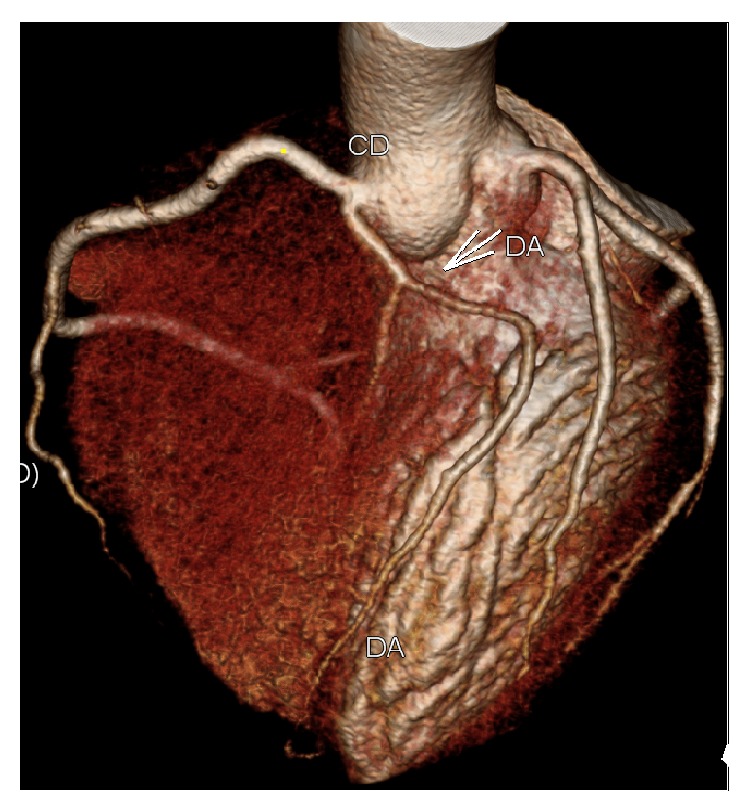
CT angiography confirming the anomalous (interarterial) course of the long LAD artery (white arrow) from the right sinus of Valsalva (close to the RCA ostium), coursing along the distal part of the AIVS (the pulmonary trunk was removed). CT, computed tomography; LAD, left anterior descending artery; RCA, right coronary artery; AIVS, anterior interventricular sulcus.
